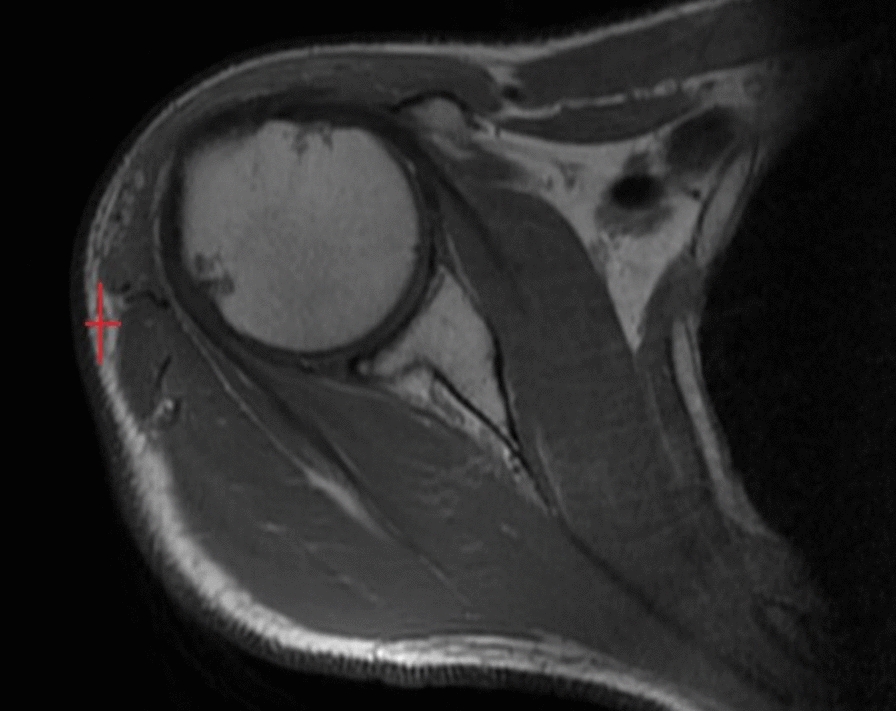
MRI after 3 weeks from RT, showing a partial radiological response, with a mass measuring 16 × 6 mm
